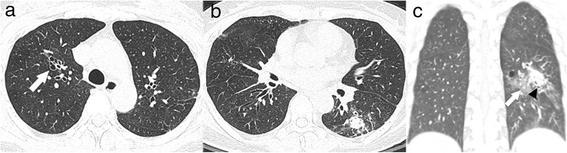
A 53-year-old woman with hemoptysis and a fungus ball. a Chest computed tomography scan showing mild bronchiectases in the right upper lobe (*arrow*) without evidence of haemorrhagic aspiration. b and c Axial chest computed tomography scan (b) and coronal reformation image (c) with a lung window setting showing an approximately 2.1 cm soft tissue nodule with the air-crescent sign (*arrow* head in c) and perilesional ground glass attenuations (*arrow*) in the superior segment of the left lower lobe (LLL)
